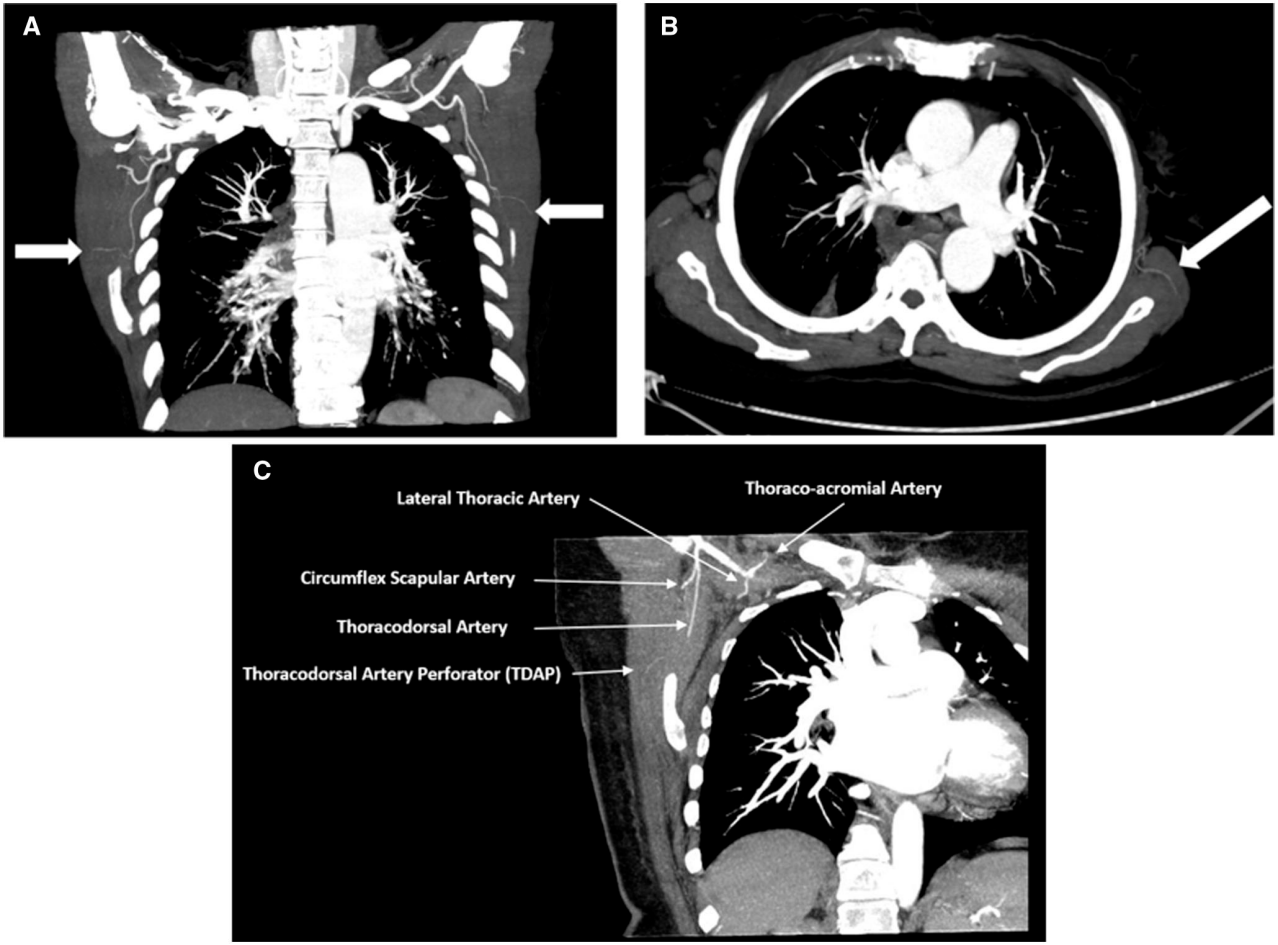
(A) CTA (maximum intensity projection, MIP) coronal view showing single TDA perforator identified bilaterally arising from the descending branch (white arrow); (B) Left TDA perforator on axial view (white arrow); (C) Branching pattern of the axillary artery: lateral thoracic artery and thoraco-acromial artery originating from the second part and subscapular artery from the third part giving off the circumflex scapular artery to continue as the TDA ending with TDA perforator.
